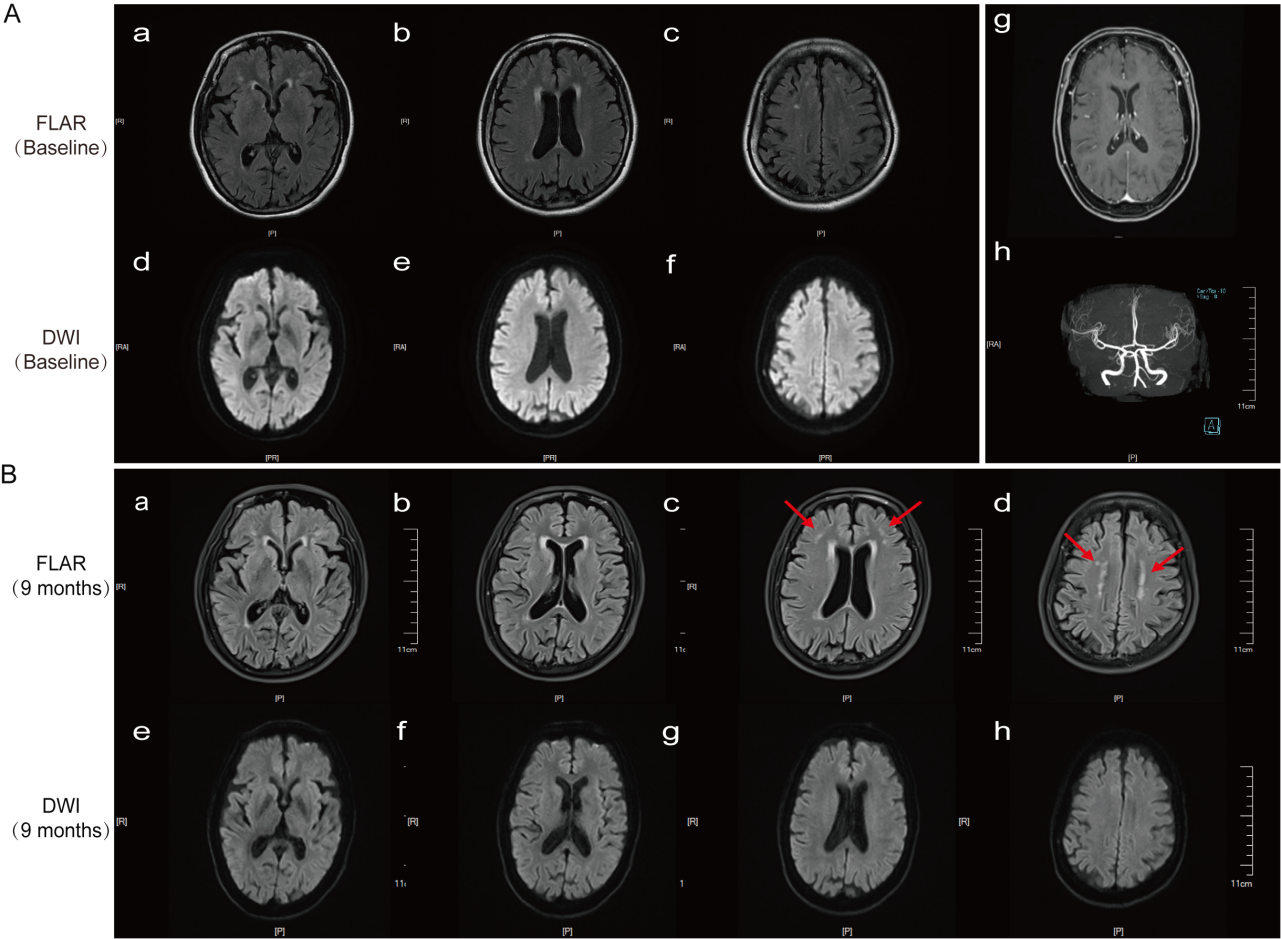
Results of cranial MRI and cerebral artery MRA on 12 June 2024, and follow-up cranial MRI on 20 March 2025. (A[a–f]) Cranial MRI (DWI) shows bilateral periventricular white matter hyperintensities (WMH) in the frontal and parietal lobes (Fazekas score = 1). (A[g]) Contrast-enhanced cranial MRI. (A[h]) Cerebral artery MRA. (B[a–h]) A follow-up cranial MRI (FLAIR) 9 months later revealed new high signal intensity at the subcortical medullary junction of the bilateral frontal lobes, along with bilateral periventricular WMH (Fazekas score = 2).
